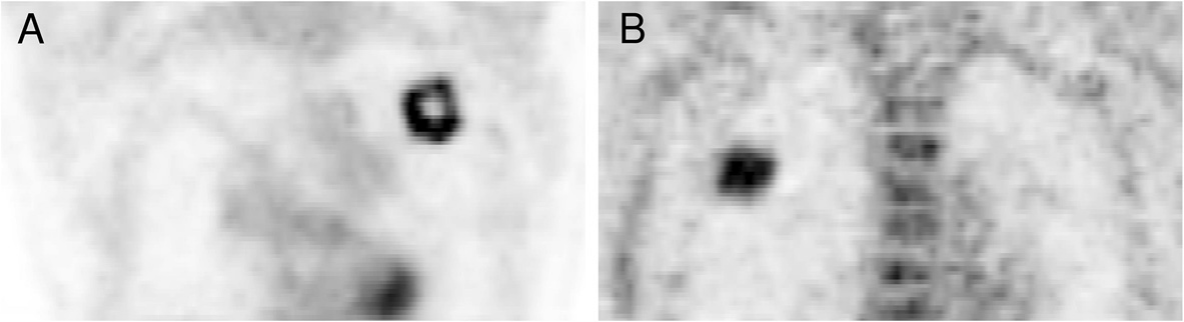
Coronal visualization of two pulmonary PET positive lesions. The lung lesion exhibiting approximately the same MTV but have different heterogeneity. The first lesion (A) has a central necrosis, leading to relatively high differences (SUVmax, 17.9%; MTV, 39.2%; TLG, 19.6%) after coregistration. For the second, rather homogeneous lesion (B), the differences are substantially lower (SUVmax, 7.4%; MTV, 7.6%; TLG, 1.4%).
